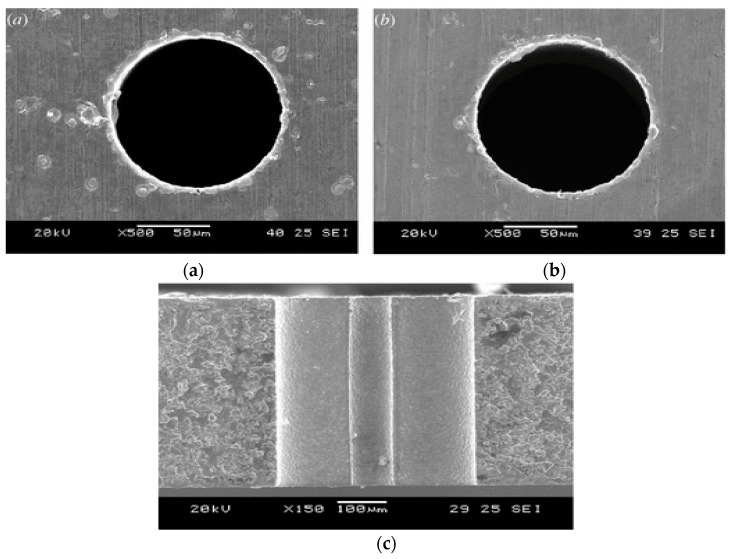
Straight micro hole machined by EDM with capacitance change and ultrasonic vibration: (a) hole entrance 117.0 µm, (b) hole exit 115.6 µm (c) Cross section of a straight micro hole machined by EDM with capacitance change and ultrasonic vibration [65]. (Adapted from [65] with permission—© 2006 Iopscience).
